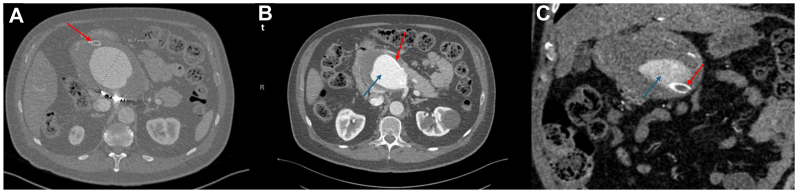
(A) Axial computed tomography (CT) image demonstrating a fusiform aneurysm arising from the common hepatic artery (CHA) with a maximal diameter of 8.6 cm (*red arrowhead* indicates the distal portion of an occluded endovascular stent). (B) Axial view showing a free-floating stent coursing along the anterior aspect of the aneurysm, with a contrast-enhanced region consistent with an endoleak (*red arrowhead* indicates the stent; *blue arrowhead* indicates the endoleak). (C) Coronal image depicting the proximal portion of the stent near the takeoff of the CHA, along with the associated endoleak (*red arrowhead* indicates the stent; *blue arrowhead* indicates the endoleak).
